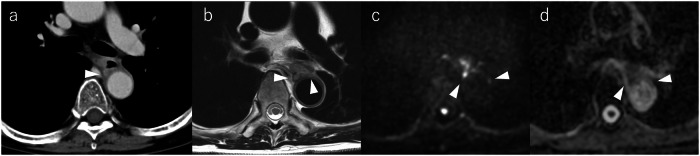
Representative case of major vascular invasion. Contrast-enhanced CT (a) shows a posteriorly protruding tumor with a Picus angle < 90°, (readers: cT3r vs. cT3br). MRI reveals a “VH-sign” on T2WI (b) plus a high signal on DWI (c), a decrease in apparent diffusion coefficient (d) was observed at the aortic interface (both readers: V-score 5). Pathology confirmed T4 vascular invasion
